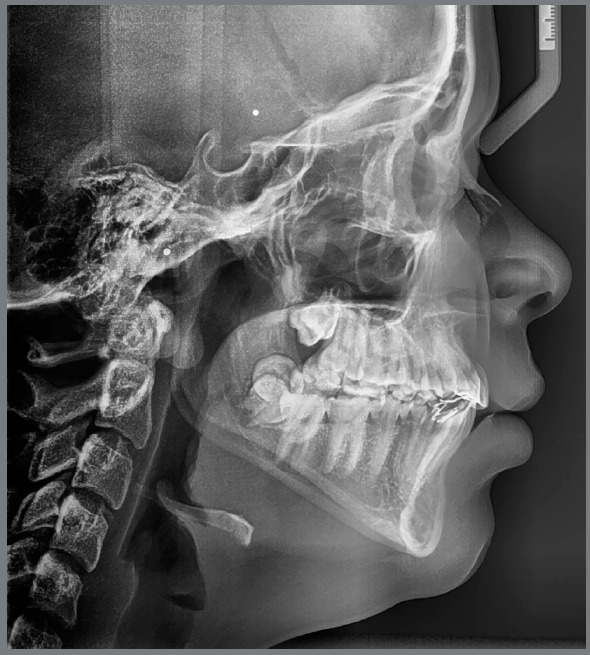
Post-treatment lateral cephalometric radiograph.
